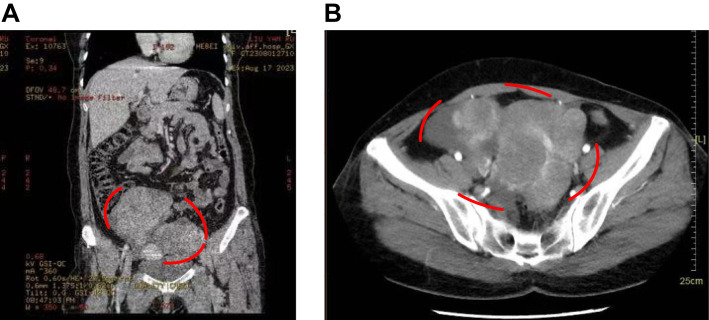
Patient’s CT images. (A) CT showed a cystic-solid masses in the adnexal area. (B) Enhanced CT indicated the marked location was a cystic-solid masses in the adnexal area, with a high possibility of malignancy.
